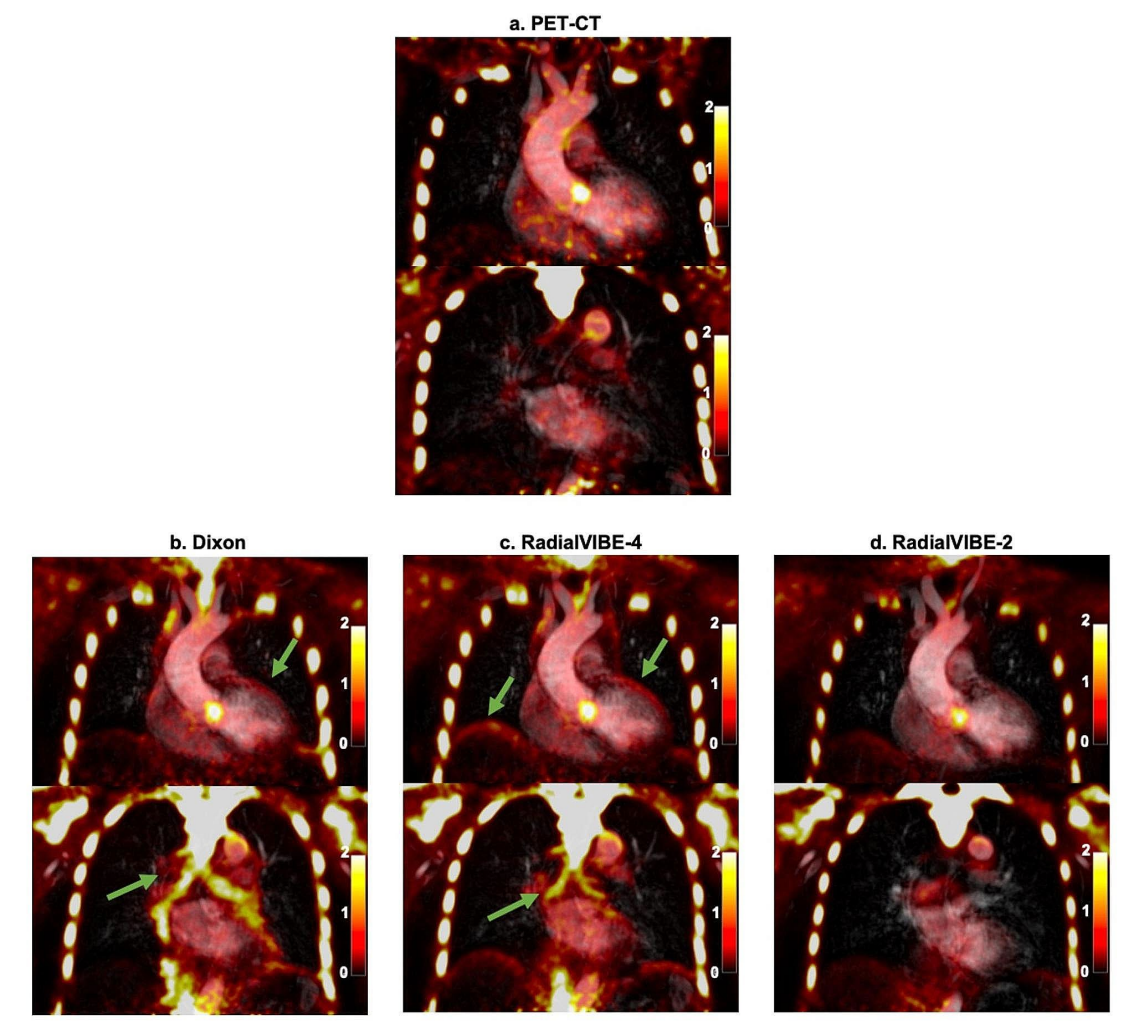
Thoracic sodium [^18^F]fluoride uptake in two coronal planes in a patient with bicuspid aortic valve. (a) Positron emission tomography (PET)-computed tomography (CT) co-registered with magnetic resonance angiography (MRA), (b) combined Dixon attenuation correction PET and MRA, (c) combined RadialVIBE-4 attenuation correction PET and MRA, and (d) combined RadialVIBE-2 attenuation correction PET and MRA. Sodium [^18^F]fluoride uptake in each PET-MRI image demonstrates a similar pattern compared with uptake in PET-CT. Note the image artefact in Dixon and RadialVIBE-4 at the bronchial tree and heart-lung and diaphragm-lung border (green arrows). Colour scale on the right of images represents standardised uptake values
